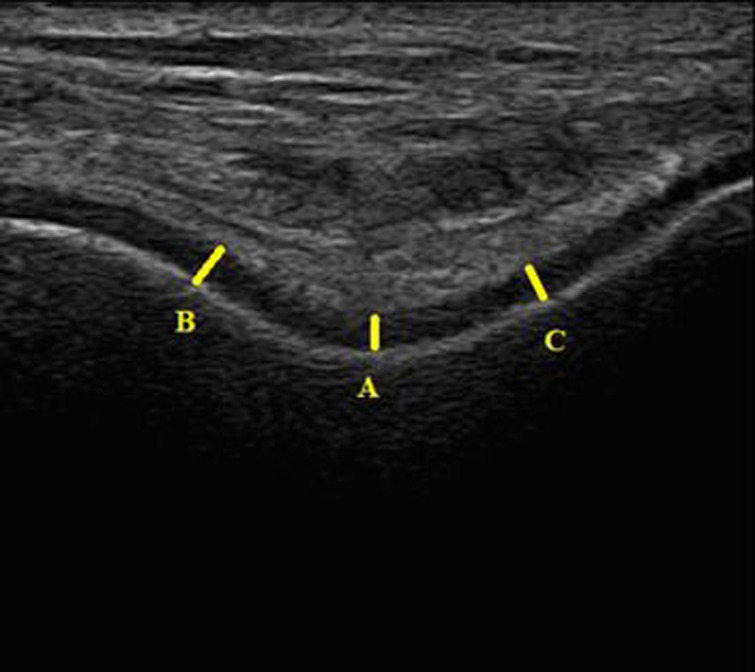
Ultrasonographic measurement of femoral cartilage thickness. A) Central (intercondylar), B) Medial, C) Lateral.
